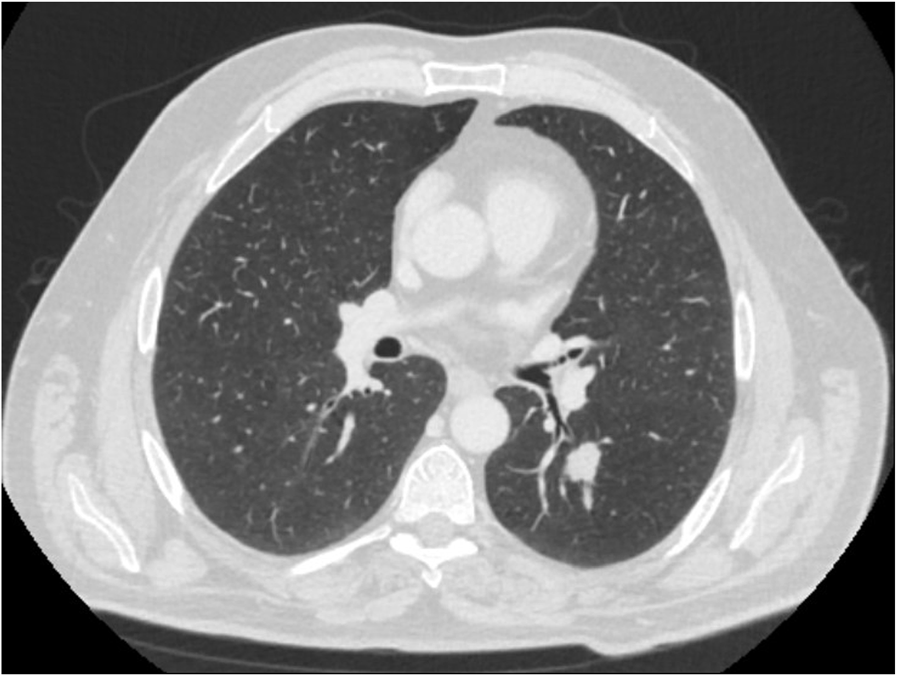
Thoracic computed tomographic scan taken in April 2016
